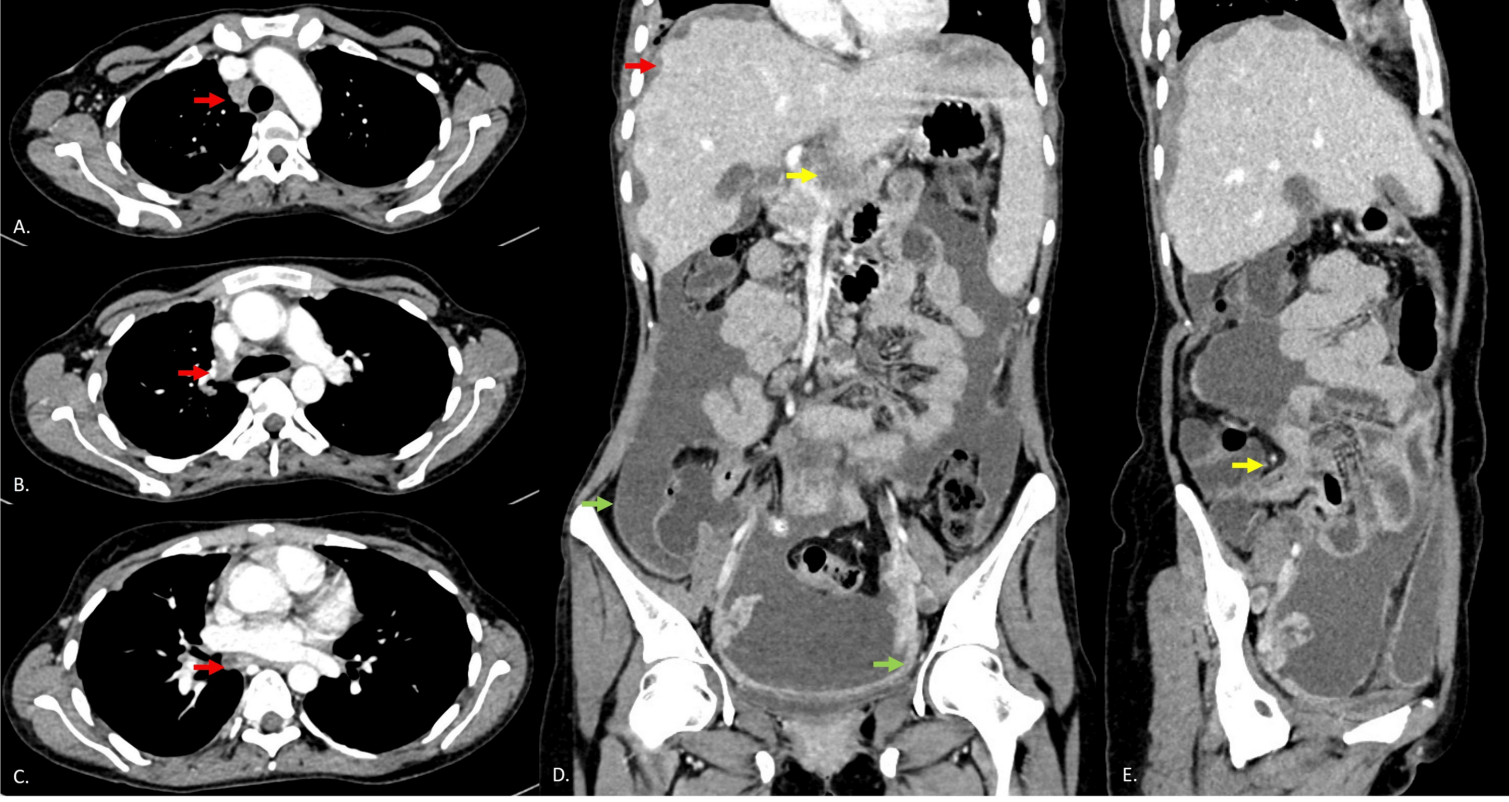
Sequential axial CECT thorax images (A)-(C) show multiple enlarged heterogeneous mediastinal lymph nodes (red arrows). (D) Additional coronal images of abdomen show scalloped appearance of liver margin (red arrow), periportal hypodense lesion infiltrating pancreas (yellow arrow) with smooth peritoneal thickening (green arrow). (E) Oblique sagittal images show thickened ileocecal junction (yellow arrow). CECT: contrast-enhanced computed tomography.
